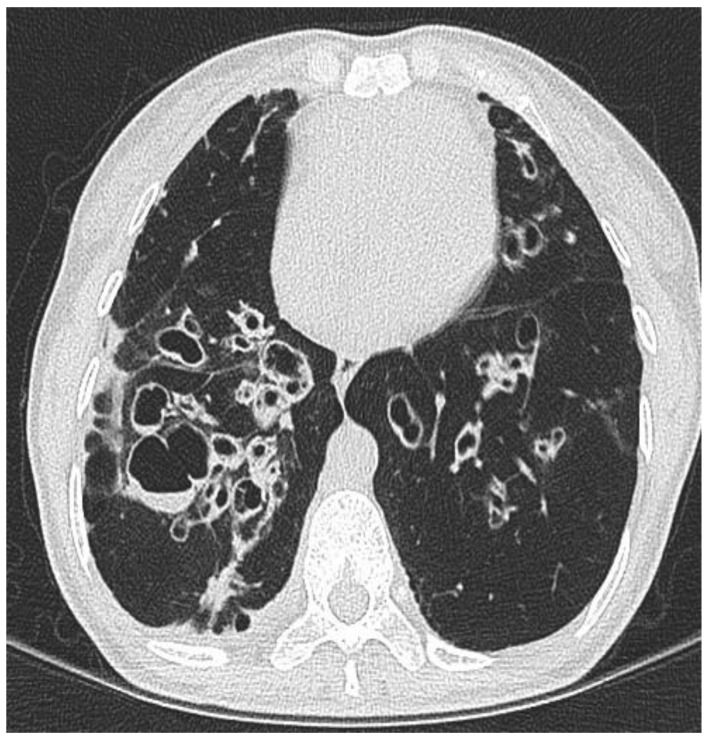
Computed tomography demonstrating cystic bronchiectasis in a patient with idiopathic bronchiectasis.
